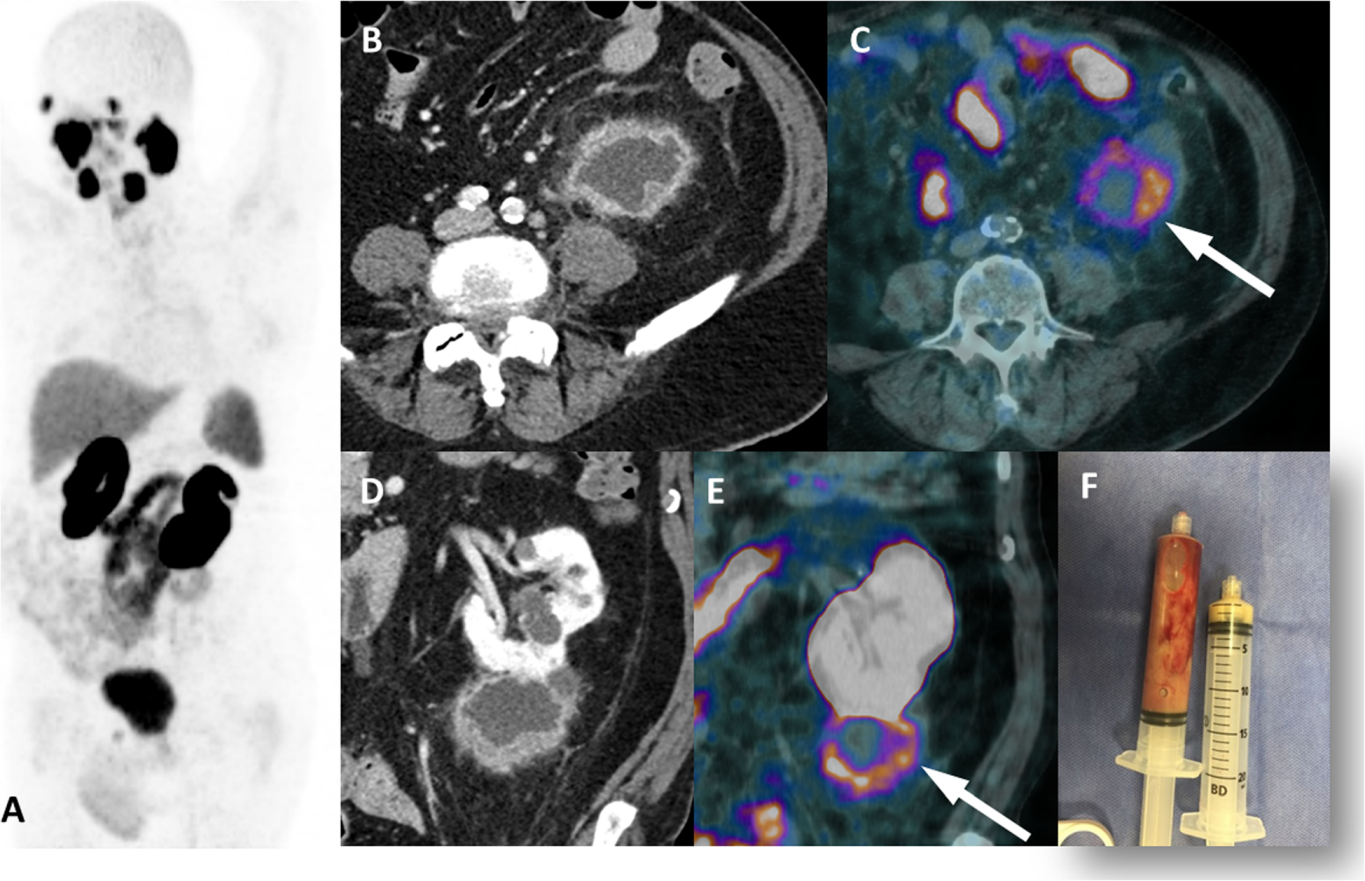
Renal abscess with PSMA uptake. ^68^Ga-PSMA-PET MIP (a), axial and coronal contrast-enhanced CT (b, c), axial and coronal PET/CT (d, e) images show a complex cystic mass on the lower pole of the left kidney presenting moderate peripheric PSMA uptake (arrows), with purulent content after percutaneous drainage (f)
